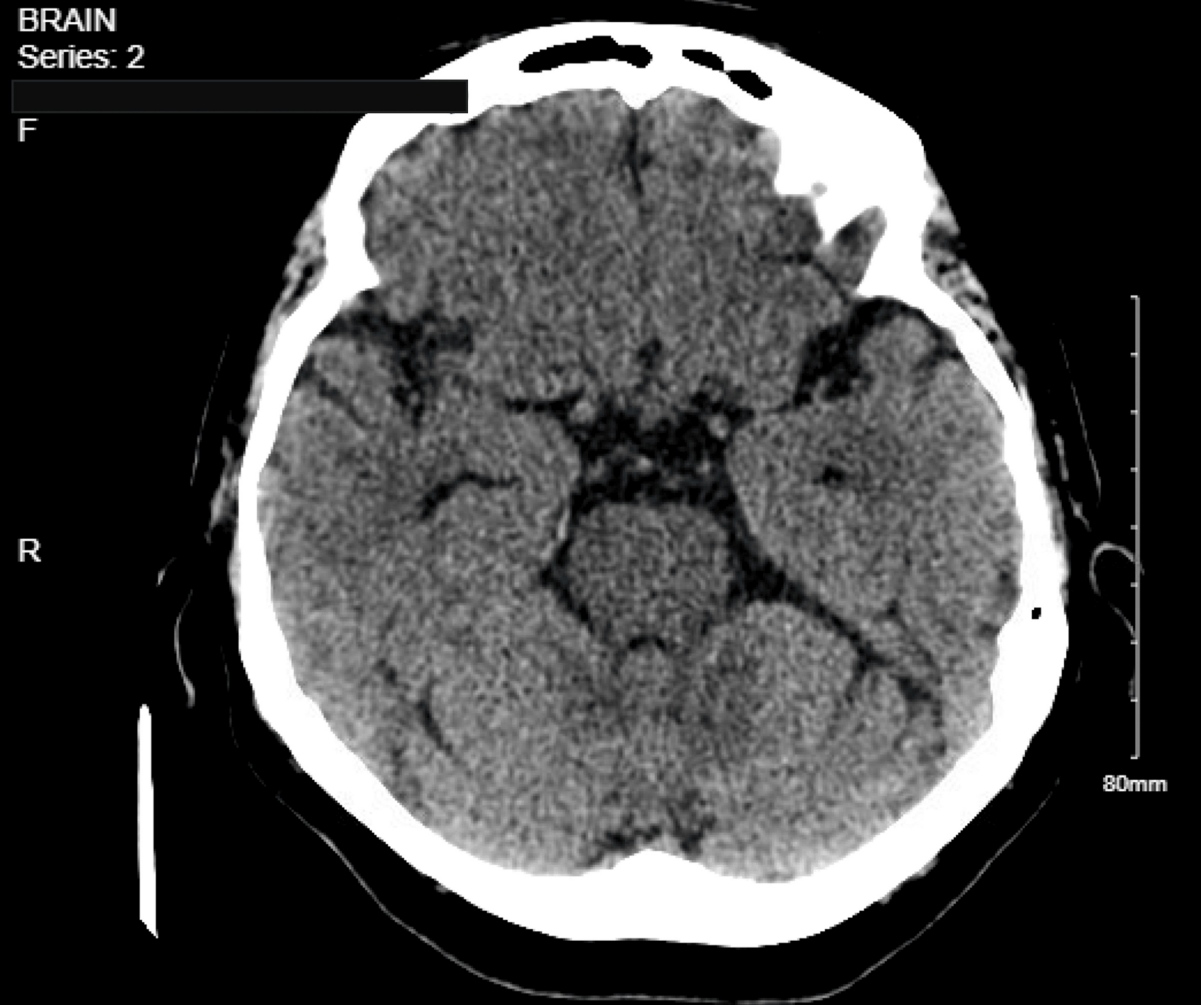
Head Computed Tomography (CT) Scan A non-contrast axial computed tomography (CT) scan of the brain demonstrates normal cerebral parenchymal attenuation without evidence of acute intracranial hemorrhage, mass effect, or midline shift. The ventricular system and cortical sulci appear within normal limits for the patient’s age. The basal cisterns are patent. No areas of abnormal hypoattenuation are identified to suggest acute ischemic infarction. The visualized portions of the posterior fossa, including the cerebellum and brainstem, are unremarkable. These findings ruled out acute brain insult that could have explained the syncopal attack.
